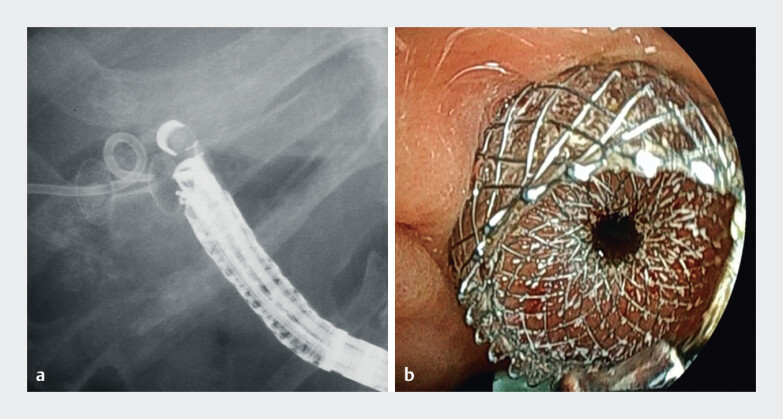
Endoscopic ultrasound-guided gallbladder drainage using a 10-mm × 10-mm Hot AXIOS.
